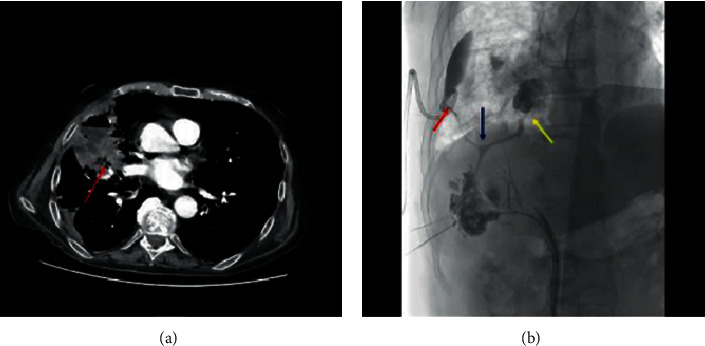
Chest-abdomen CT scan showing a 50 mm-diameter abscess of the middle lobe communicating with the subsegmental bronchi (arrow) (a); percutaneous cholangiography revealing a “Y”-shaped fistula originating from intrahepatic bilioma (blue arrow) and divided in two arms which reached separately the pleural space (red arrow) and the middle lobe abscess up to one segmental bronchus (yellow arrow) (b).
